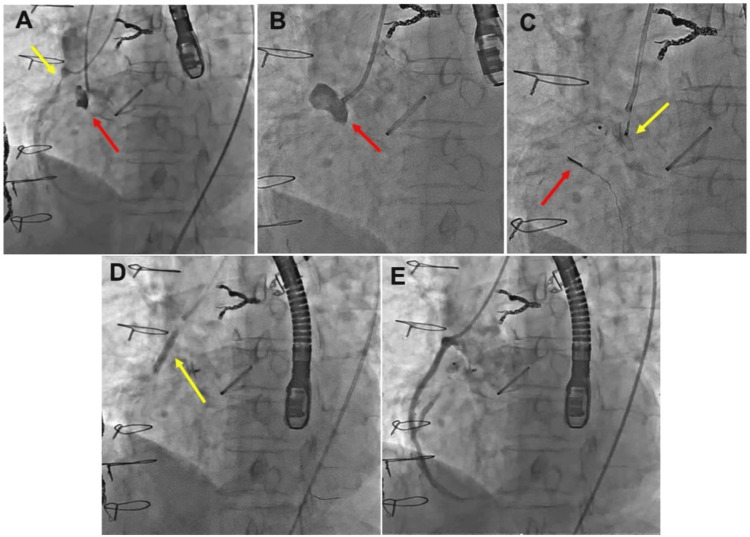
(A) Simultaneous angiography to delineate the anatomy, showing 90% stenosis of the SVG (yellow arrow), aortic pseudoaneurysm (red arrow), and the anatomy of the mechanical valve. (B) Angiography to evaluate the morphology of the aortic pseudoaneurysm (red arrow). (C) Placement of the AVP II 9/12 mm (yellow arrow) under ICE guidance (red arrow). (D, E) Stent placement and patency in the SVG stenosis. SVG: saphenous vein graft, AVP II: Amplatzer Vascular Plug II, ICE: intraoperative intracardiac echocardiography
